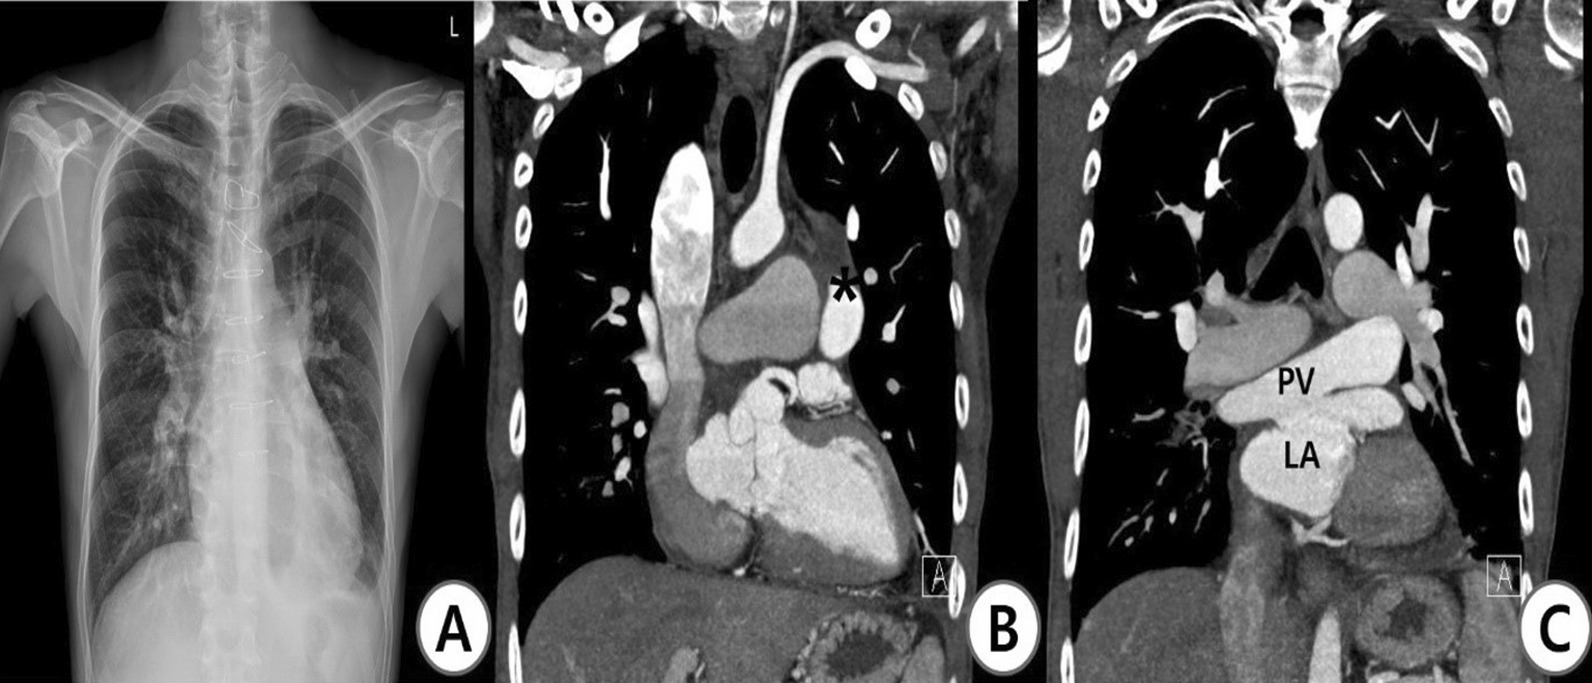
Postoperative computed tomography (CT). A Chest X-ray at discharge. B, C Coronal view on chest CT at 2.5 years after surgery. The PV was connected to the LA. PV, pulmonary vein; LA, left atrium. *ligated vertical vein
